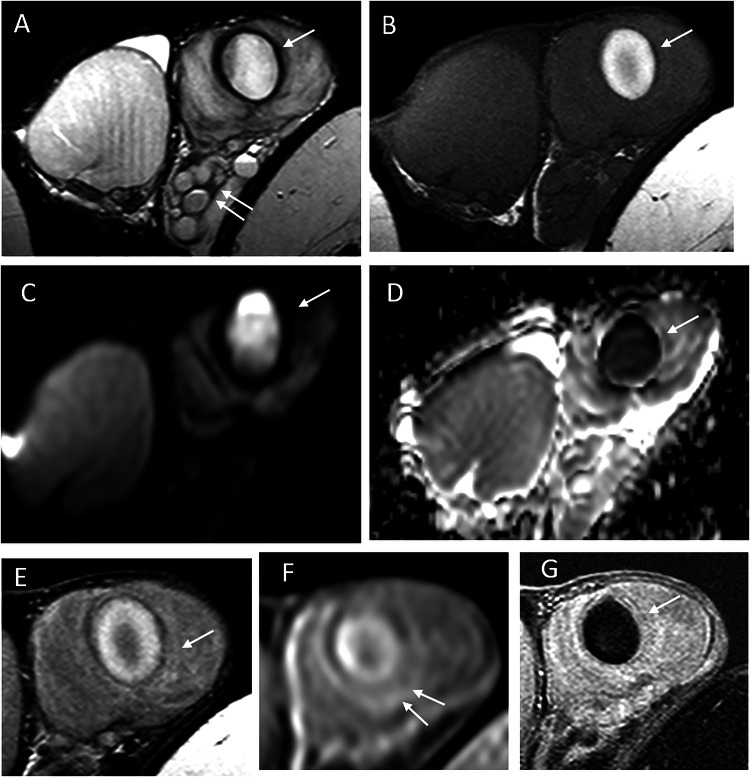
Scrotal MRI of the same patient, performed 28 days after symptom onset, is consistent with a late subacute hematoma. A T2-weighted image showing a hyperintense lesion with a hypointense rim (arrow), consistent with hemosiderin deposition; dilated scrotal veins are visible (double arrows). A striated pattern of the adjacent parenchyma is noted. B Predominant hyperintensity on T1-weighted images, with areas of lower signal intensity corresponding to extracellular methemoglobin. C Diffusion-weighted imaging (high b-value) showing hyperintensity, with corresponding hypointensity on the ADC map (D). E Post-contrast T1-weighted image showing a striated enhancement pattern of the parenchyma, making assessment of lesion enhancement difficult. F Early dynamic contrast-enhanced sequence showing slightly increased enhancement of the adjacent parenchyma. G Subtraction image confirming the absence of internal lesion enhancement
